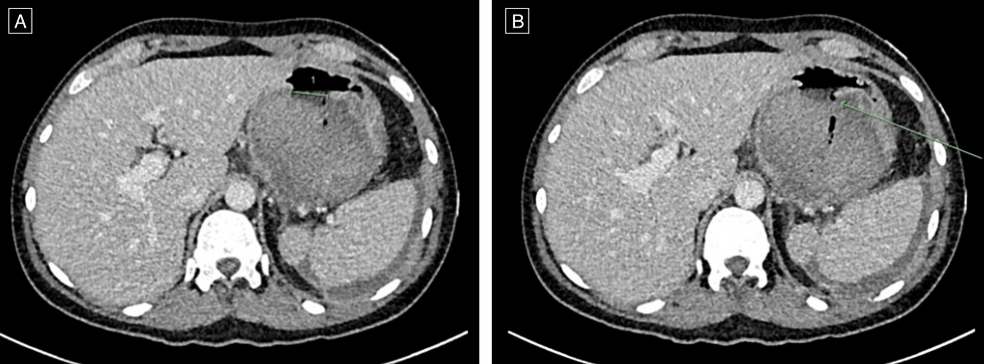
CT abdomen demonstrating interval decompression of the large pancreatic pseudocyst. (A) A new 25 mm defect is demonstrated involving the posterior wall of the stomach. (B) Internal gas locules within the lobulated pseudocyst are in keeping with a contained perforation and subsequent infected pseudocyst. CT: computer tomography.
